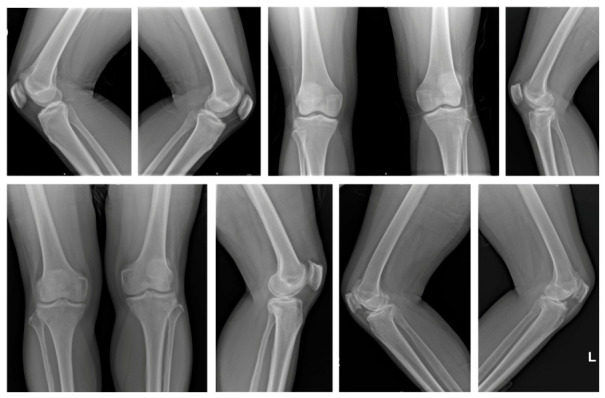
Representative samples of knee X-ray images from the MedUP Intelligence Healthcare and Data Innovation Center, School of Medicine, University of Phayao. The images demonstrate variability in anatomical structures, imaging orientations (anterior–posterior and lateral views), and bone density patterns across the three classes: Normal, Osteopenia, and Osteoporosis. These variations highlight the complexity of the dataset and the necessity for robust feature extraction models.
